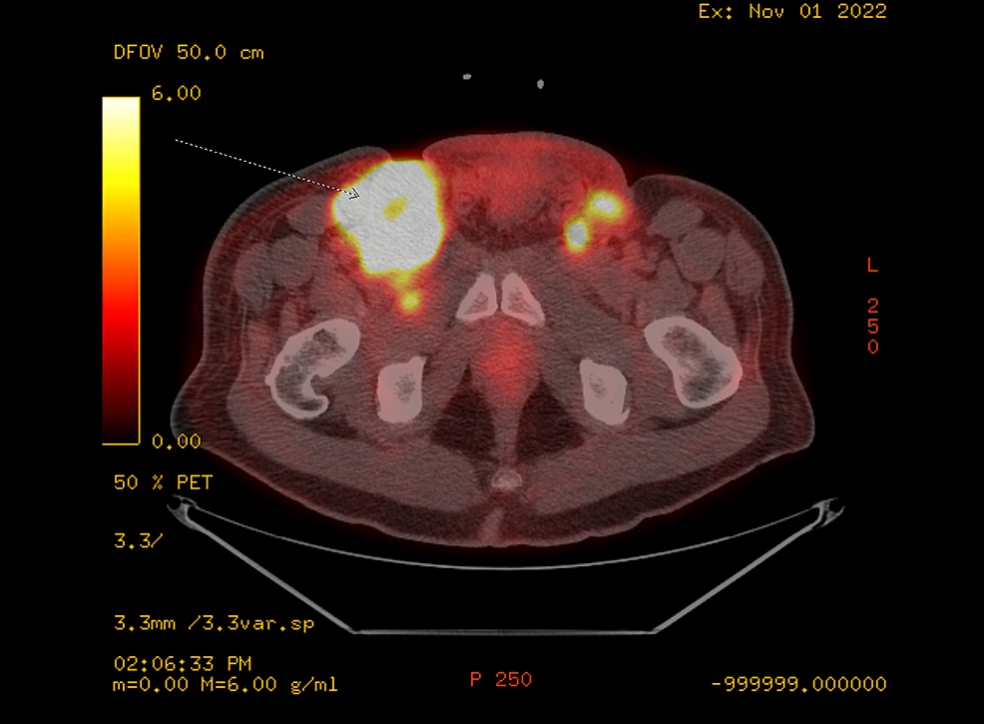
Post-chemotherapy PET/CT demonstrating disease progression in inguinal regions Arrow pointing at bulky refractory mass in right inguinal region.
